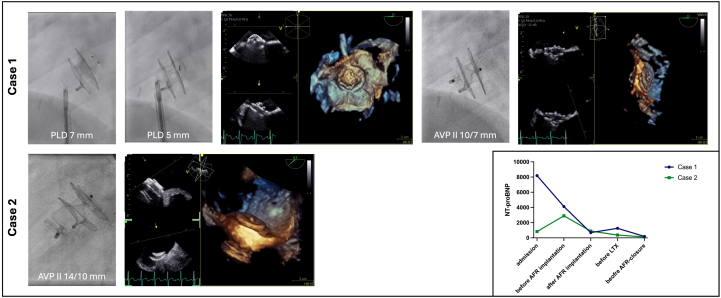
Closure of the Atrial Flow Regulator Fluoroscopic images and simultaneous 2D and 3D transesophageal echocardiography demonstrate (Top Row) attempted closure with paravalvular leak devices and final closure with the AVPII in case 1 and (Bottom Row) closure with the AVPII in case 2. (Bottom Right) Graph showing NT-proBNP levels at different time points: admission, before and after AFR implantation, before lung transplantation, and before AFR closure. 2D = two-dimensional; 3D = three-dimensional; AFR = atrial flow regulator; AVPII = Amplatzer vascular plug II; LTX = lung transplantation; NT-proBNP = N-terminal pro–B-type natriuretic peptide; PLD = paravalvular leak device.
